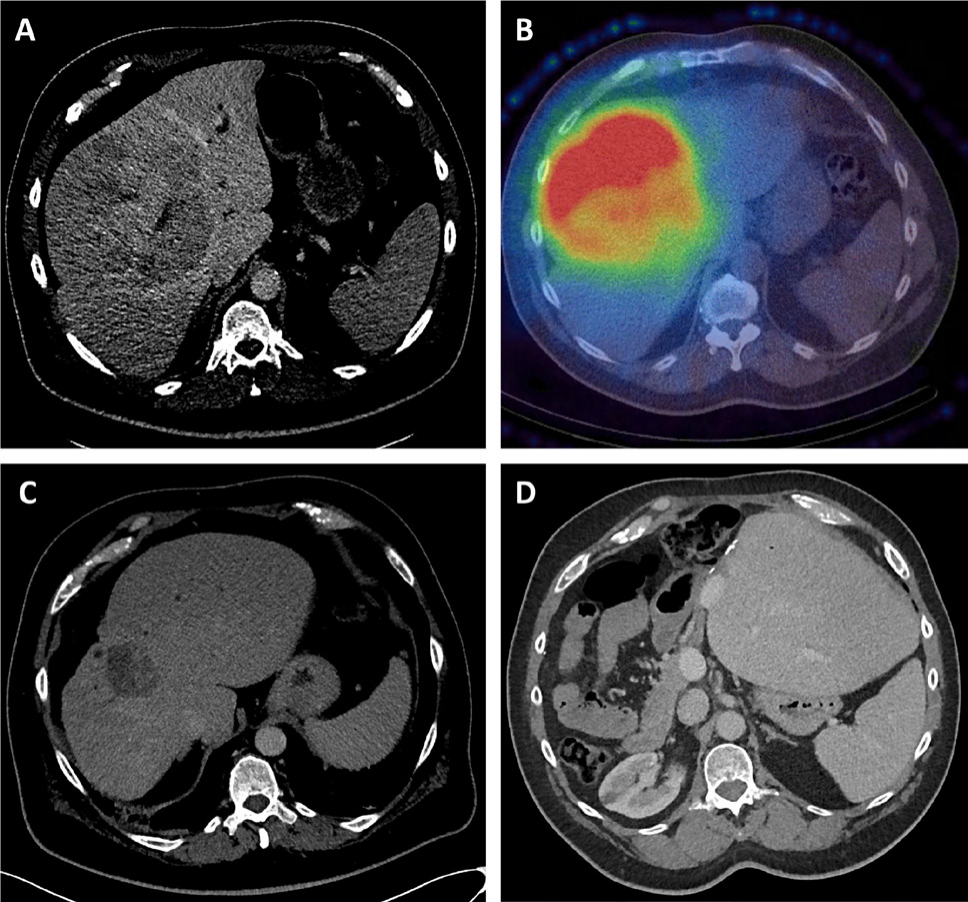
A selective internal radiation therapy (SIRT) case study. A 56-year-old non-cirrhotic patient had a 14 cm moderately differentiated hepatocellular carcinoma in the right liver lobe and segment 4, with a small left lateral lobe (future liver reminant (FLR)) (A). Single-photon emission computed tomography (CTSPECT) post SIRT demonstrated high uptake of SIRT within the tumour (B). CT scan at 18 months, following a second SIRT delivering 350 Gy to the tumour, demonstrated a reduction in tumour volume (from 1695 cc to 63 cc) with hypertrophy of the FLR (C), following which a curative resection was performed. CT scan 5 years later confirmed the patient remains free of tumour recurrence.
